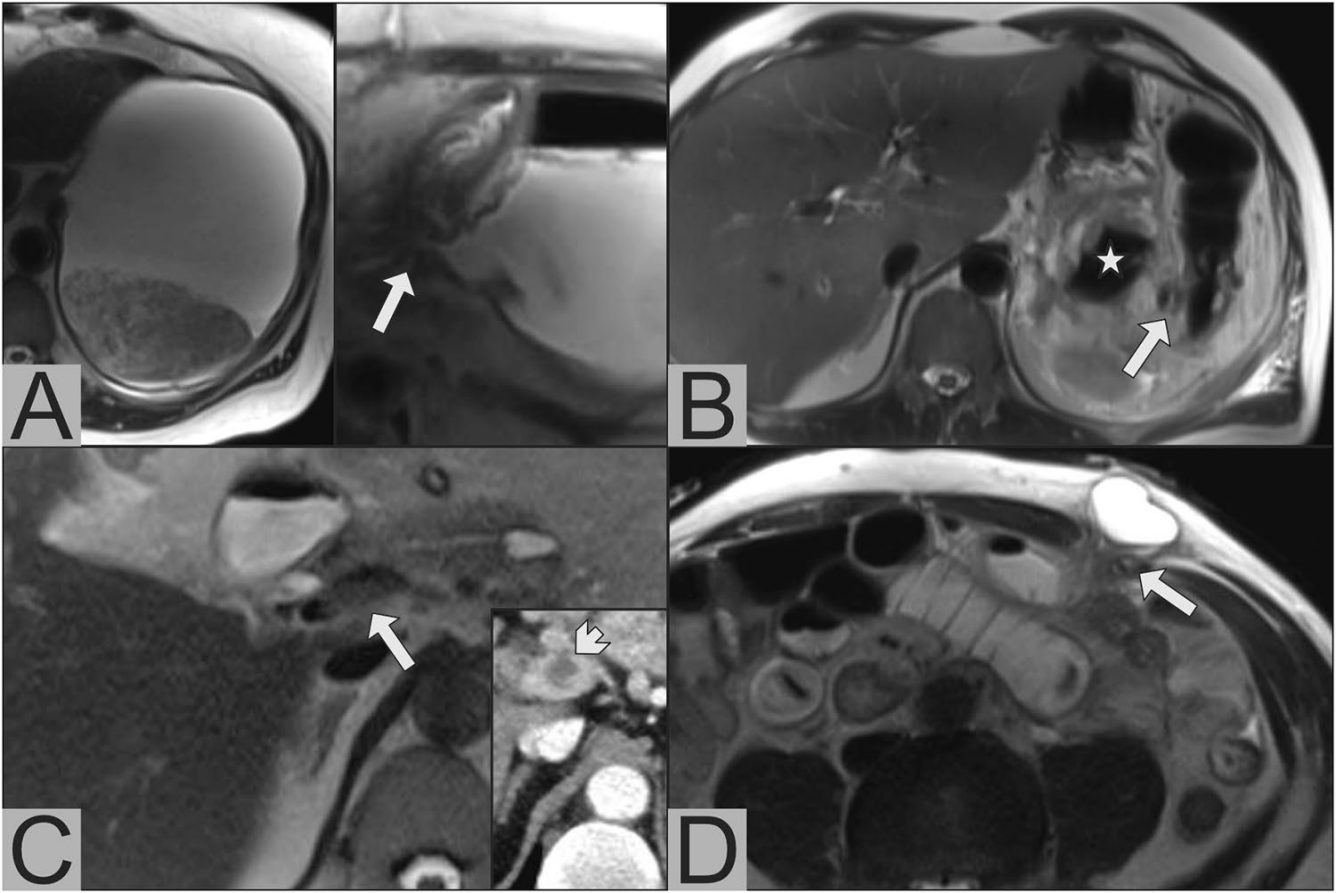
Important additional findings in PoSSe MRI; a Anastomotic stenosis of the gastrojejunostomy. Dilated and fluid-filled stomach on the left side. On the right side, narrow anastomosis (arrow) with prestenotic dilatation. b Bowel perforation. Perforated diverticula (arrow) of the left hemicolon with adjacent free gas in the peritoneal cavity (asterisk), these imaging findings were confirmed by subsequent surgery. c Partial portal vein thrombosis, characterized by missing flow void in PoSSe MRI (arrow), confirmation of thrombosis in ceCT (arrowhead). d Incarcerated hernia with a small hernial orifice (arrow)
